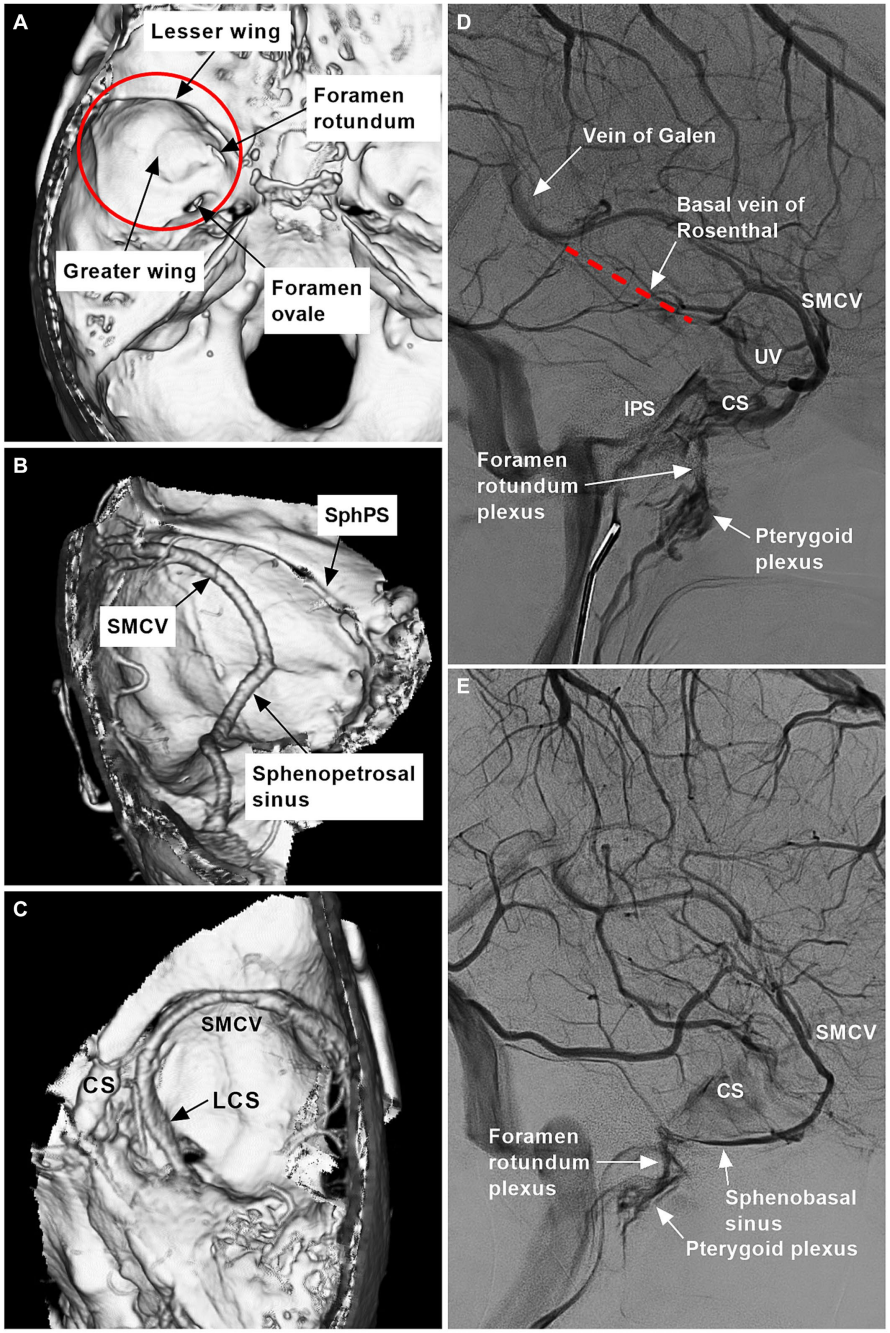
Range and venous structures of the sphenoidal region. (A) Reconstructive three-dimensional CT image showing the sphenoidal region (red circle) and bony structures (arrows). (B) CTA image showing the SphPS, SMCV, and sphenopetrosal sinus (arrows). (C) CTA image showing the LCS (arrow), CS, and SMCV. (D) DSA image showing the vein of Galen, the basal vein of Rosenthal (red dotted line), the foramen rotundum plexus, the pterygoid plexus (arrows), the IPS, the CS, the SMCV, and the UV. (E) DSA showing the foramen rotundum plexus, pterygoid plexus and sphenobasal sinus (arrows), CS, and SMCV. CS, cavernous sinus; CT, computed tomography; CTA, CT angiography; DSA, digital subtraction angiography; IPS, inferior petrous sinus; LCS, laterocavernous sinus; SMCV, superior middle cerebral vein; SphPS, sphenoparietal sinus; UV, uncal vein.
